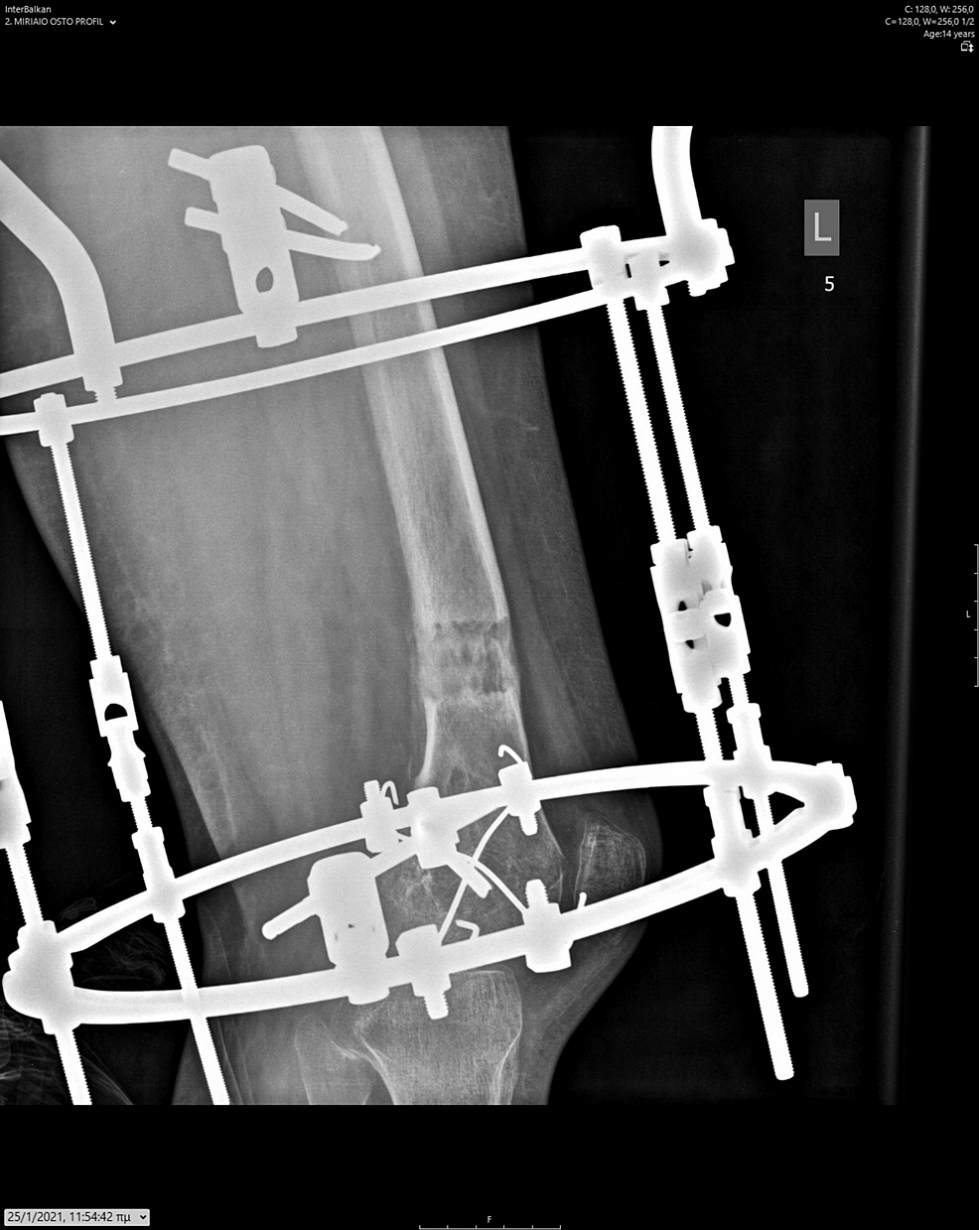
Lateral X-ray at the conclusion of the procedure, displaying appropriate new bone formation.
